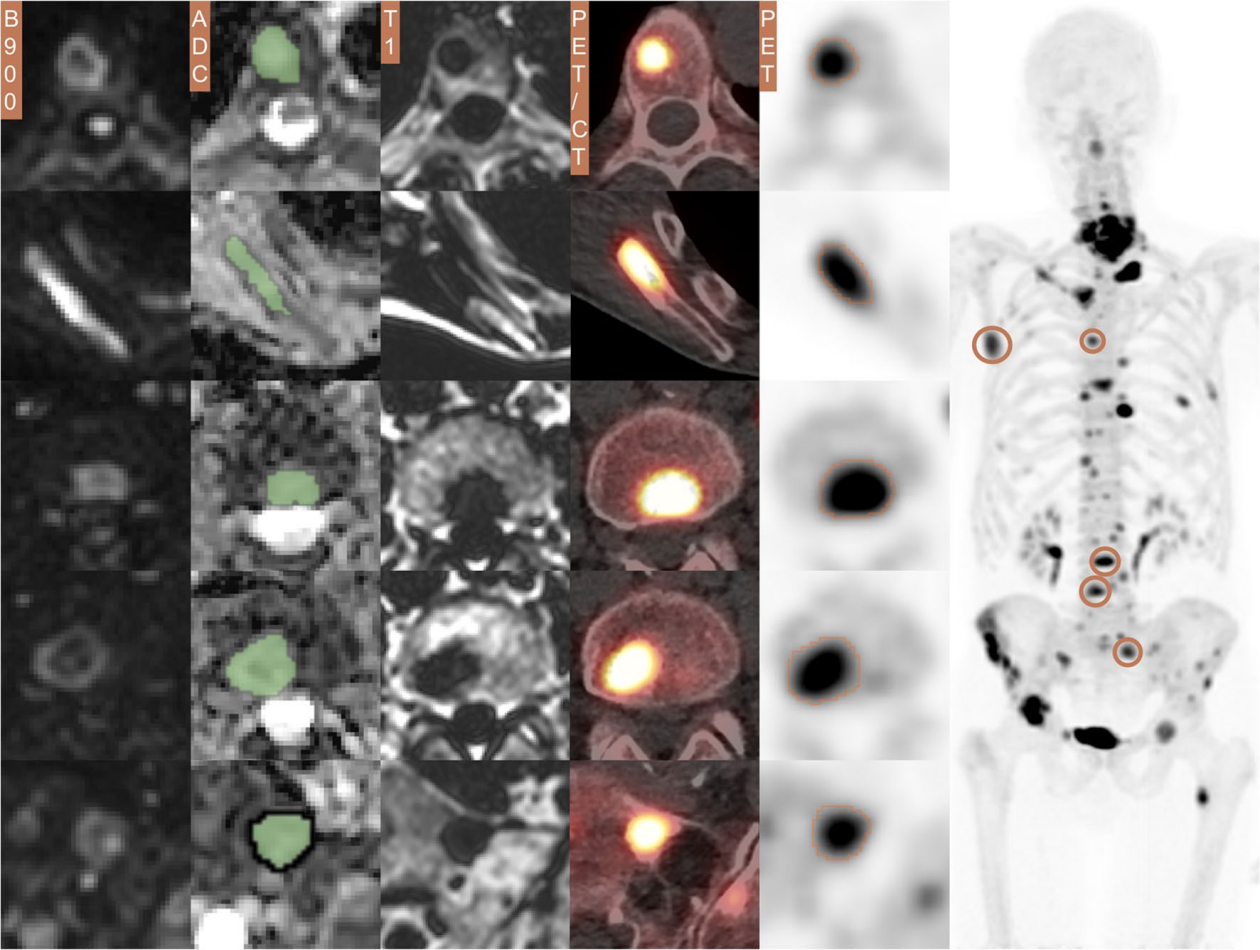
Target lesion measurements in a 65-year-old metastatic castrate-resistant prostate cancer patient, 5 target lesions on *b*900 DWI, ADC (with green segmentation), T1-weighted and 18F-NaF PET/CT fusion, and PET (delineated) images; apparent difference in lesion size between DWI and PET images may relate to the difference in functional properties used to generate image contrast, variance in patient positioning, and slice selection as well as difference in resolution of these two imaging techniques
